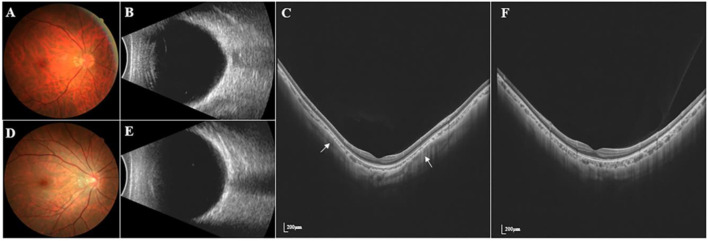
Fundus photography, B-ultrasound and SS-OCT images of PS and NPS. (A–C) Right eye images of a 39-year-old male with PS, whose AL was 28.96 mm. (D–F) Right eye images of a 28-year-old male without PS, whose AL was 28.66 mm. The white arrow points to the edge of the PS. The thickness of the choroid was the thinnest at the edge of the staphyloma, being thickened gradually from the edge to the periphery and the posterior pole of the fundus.
